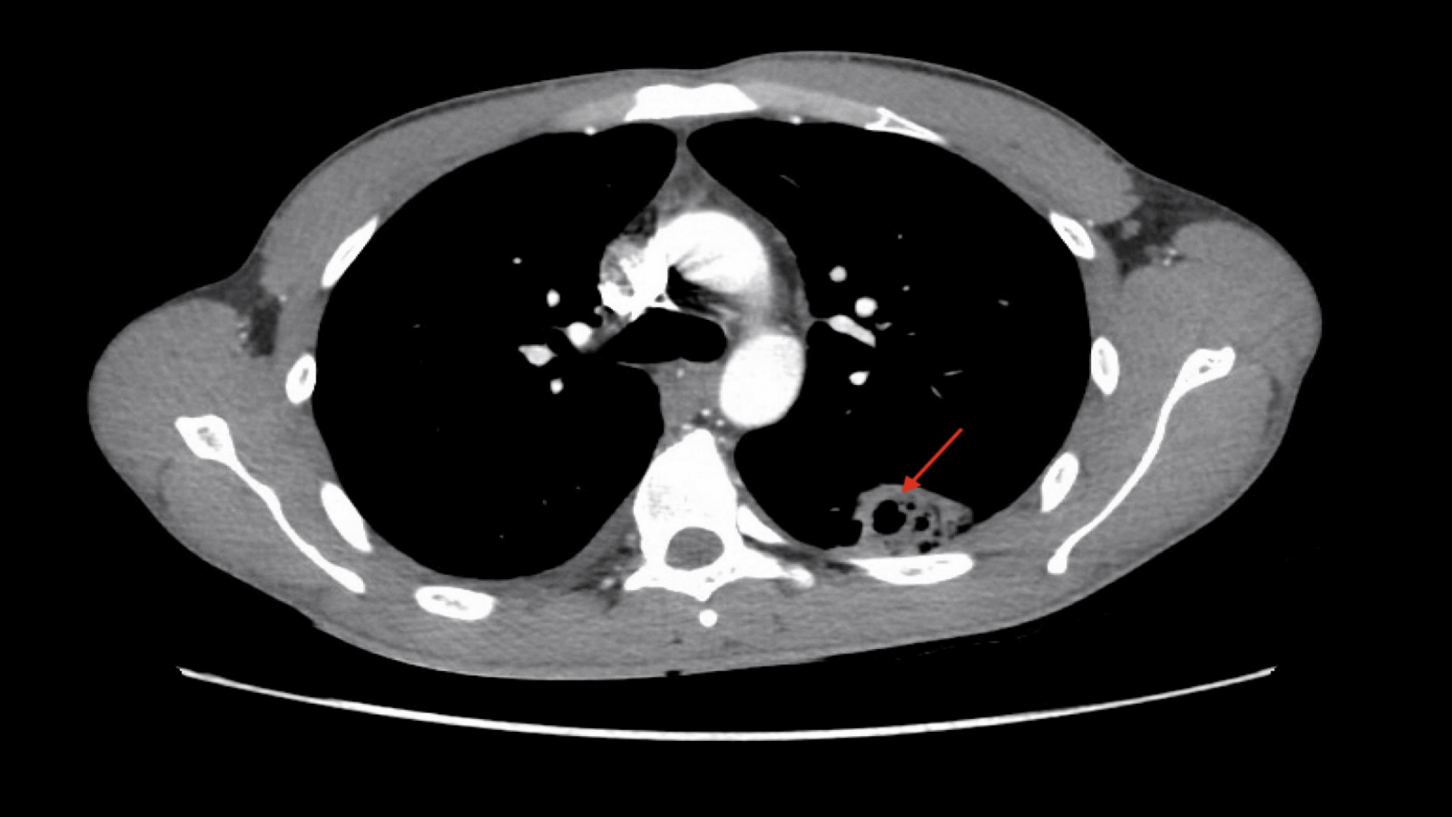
Red arrow showing cavitary lesion in the lung base. The CT scan revealed multiple discrete cavitating lesions (as shown by the red arrow) in both lungs, accompanied by several small non-cavitating lesions. Additionally, there were moderate-sized pleural effusions bilaterally, with multiple loculations within the pleural effusions.
